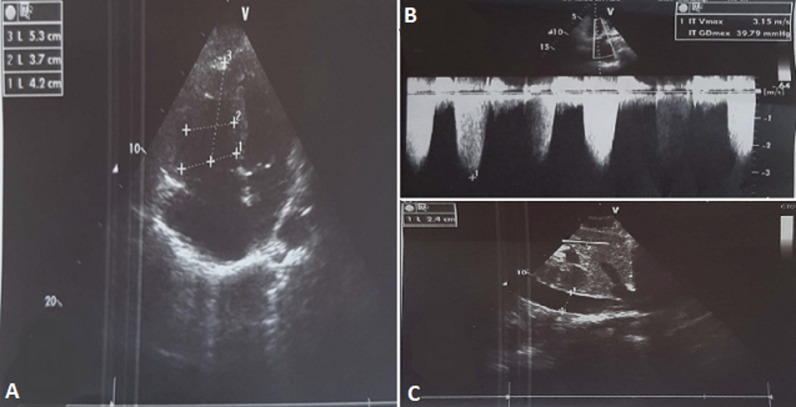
transthoracic ultrasound showing: A) right ventricular dilation; B) tricuspid insufficiency flow; C) dilated lower vena cava
